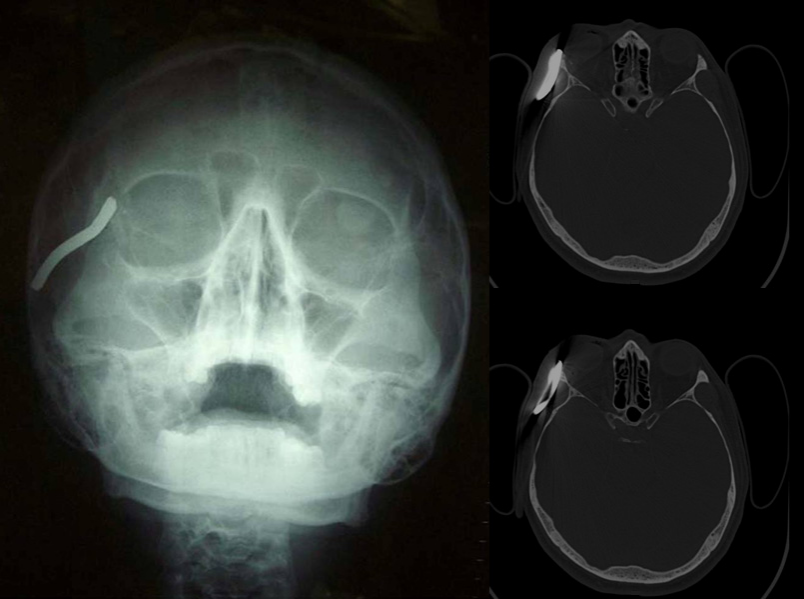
Cranial X-rays and some axial sections of the computed tomographic scan.
